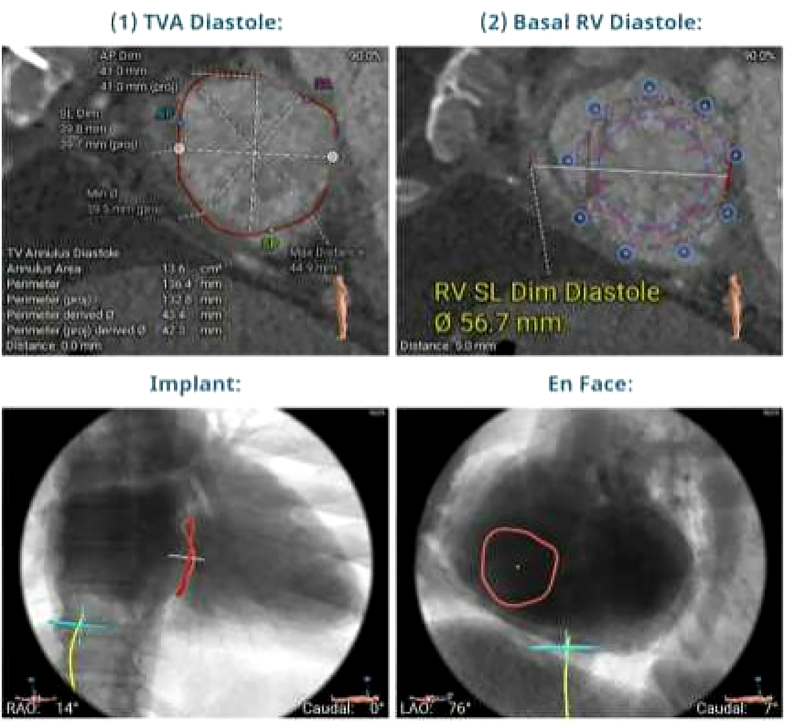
Computed Tomography Analysis for Transcatheter Tricuspid Valve Replacement RV = right ventricle; TVA = tricuspid valve atresia.
